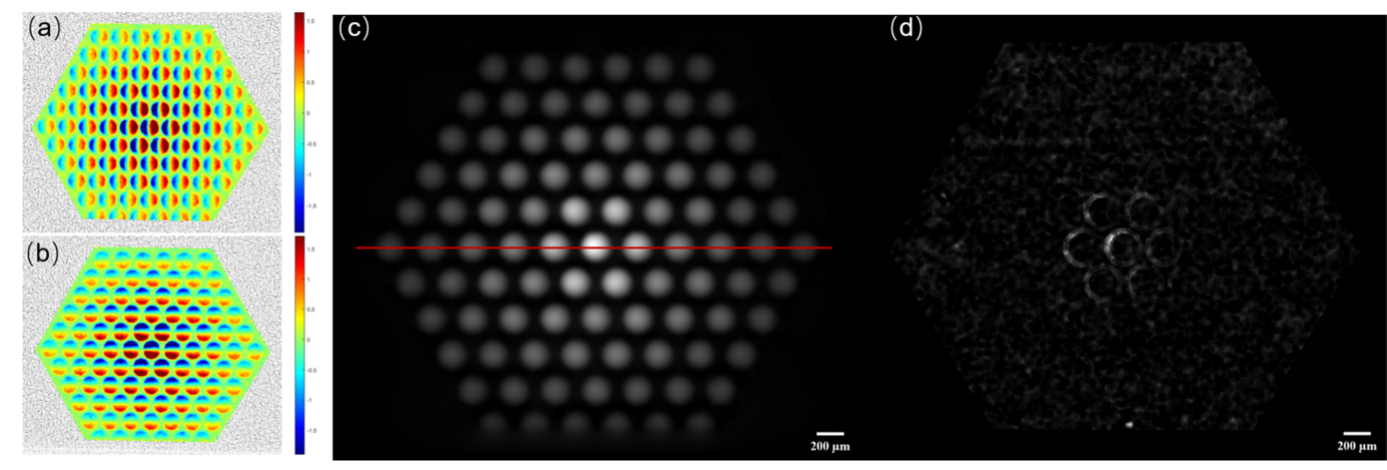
Imaging metrology of the micro-lens array by DLD-STXI. (*a*) The *u* displacement map of speckle tracking X-ray imaging, (*b*) the corresponding *v* displacement map, (*c*) phase image and (*d*) dark-field image.
